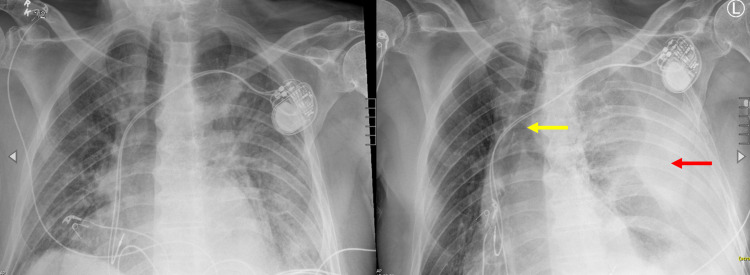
Left: Initial chest X-ray immediately after procedure. The film shows good lead position and mild, bilateral lung field infiltrates with possible small left-sided pleural effusion. Right: Emergent repeat chest X-ray showing newly appeared complete opacification of the left hemithorax (red arrow) with left lung collapse. Rightward mediastinal shift is present, as evidenced by the right-sided carina (yellow arrow).
